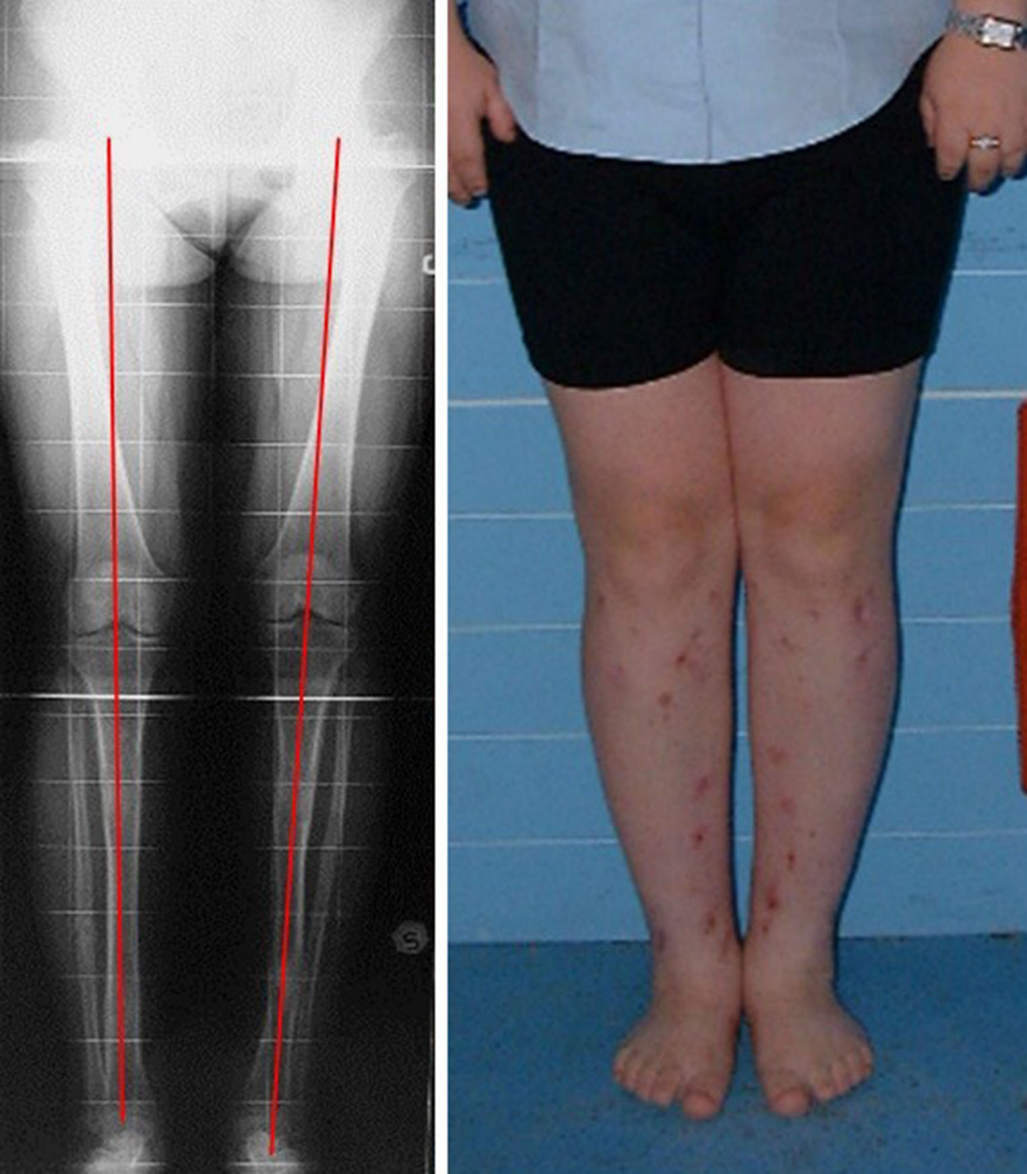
X-ray after frame removal and photograph of patient
